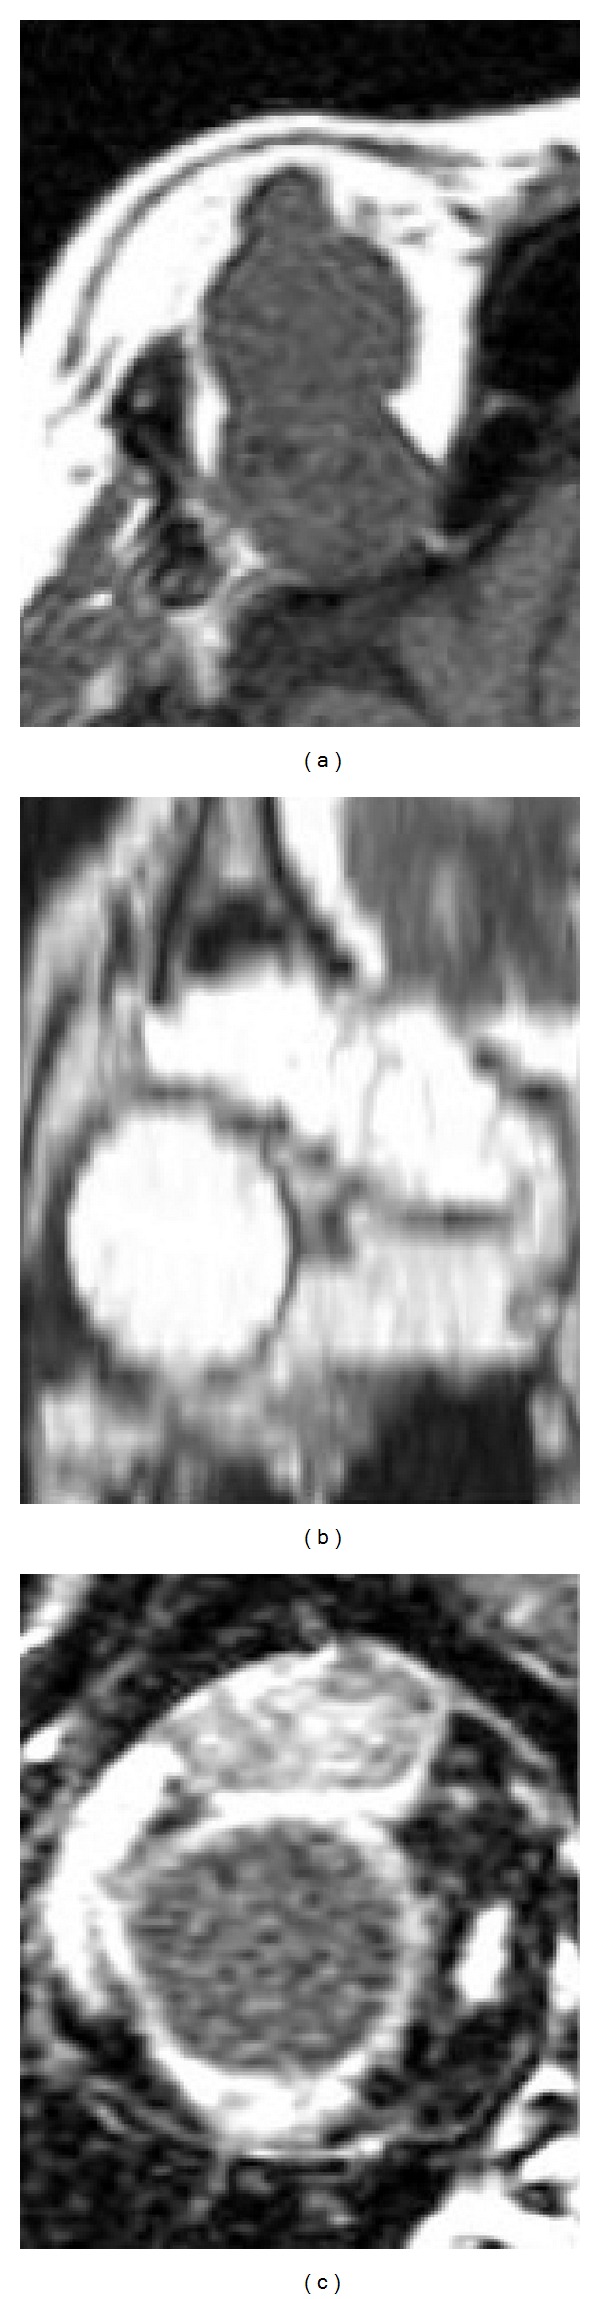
Axial magnetic resonance imaging (MRI) revealed that the lesion was located in the extraconal region, just above the levator palpebral muscle; the mass was found to be isointense on T1-weighted image (a) and to have hyperintensity on T2-weighted image (b). (c) Coronal MR image after fat suppression demonstrated marginal enhancement of the cystic mass.
